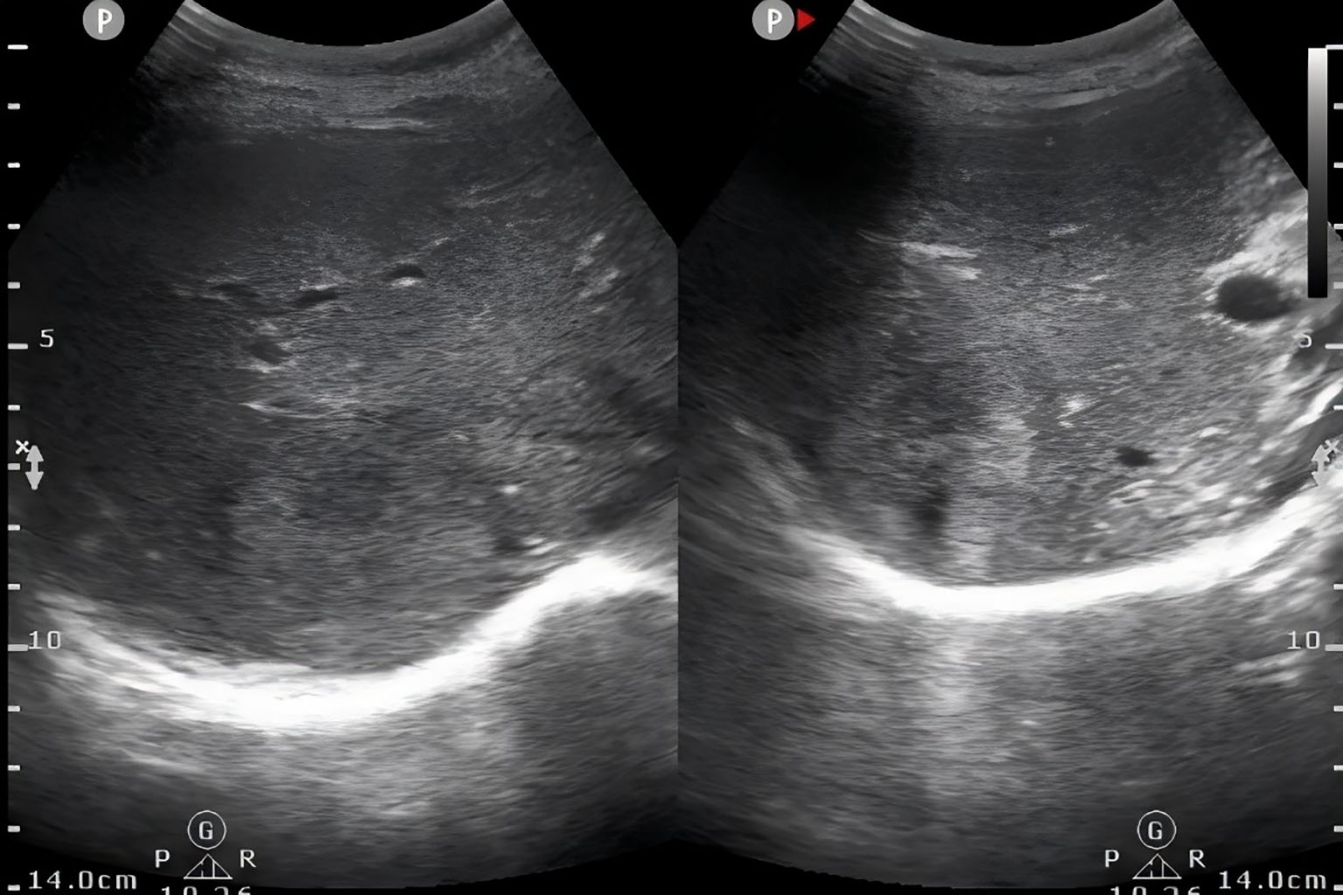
Abdominal ultrasonography showed heterogeneous hypoechoic nodules in right lobe of liver (S7,8).
